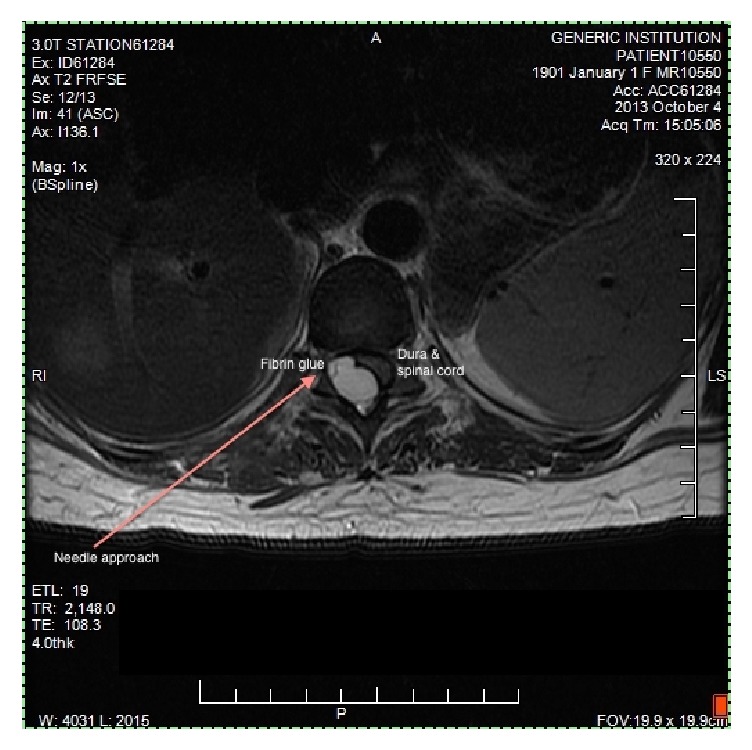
Axial T2 postinjection MRI demonstrating extradural hydrogel sealant deposition with leftward displacement of the thecal sac and spinal cord at T10-11.
